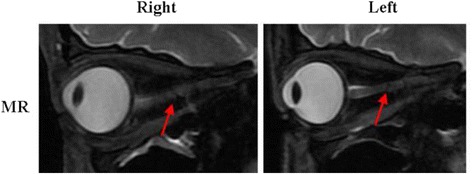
Optic nerves on MRI, showing that bilateral optic nerves are injured (red arrows)
